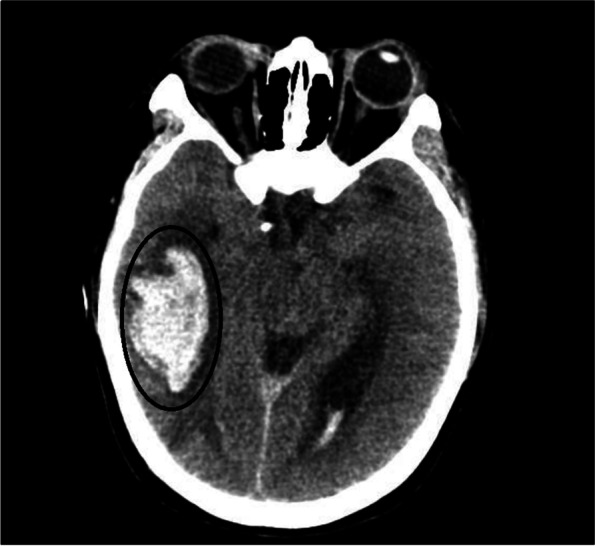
CT scan of cerebral hemorrhage
